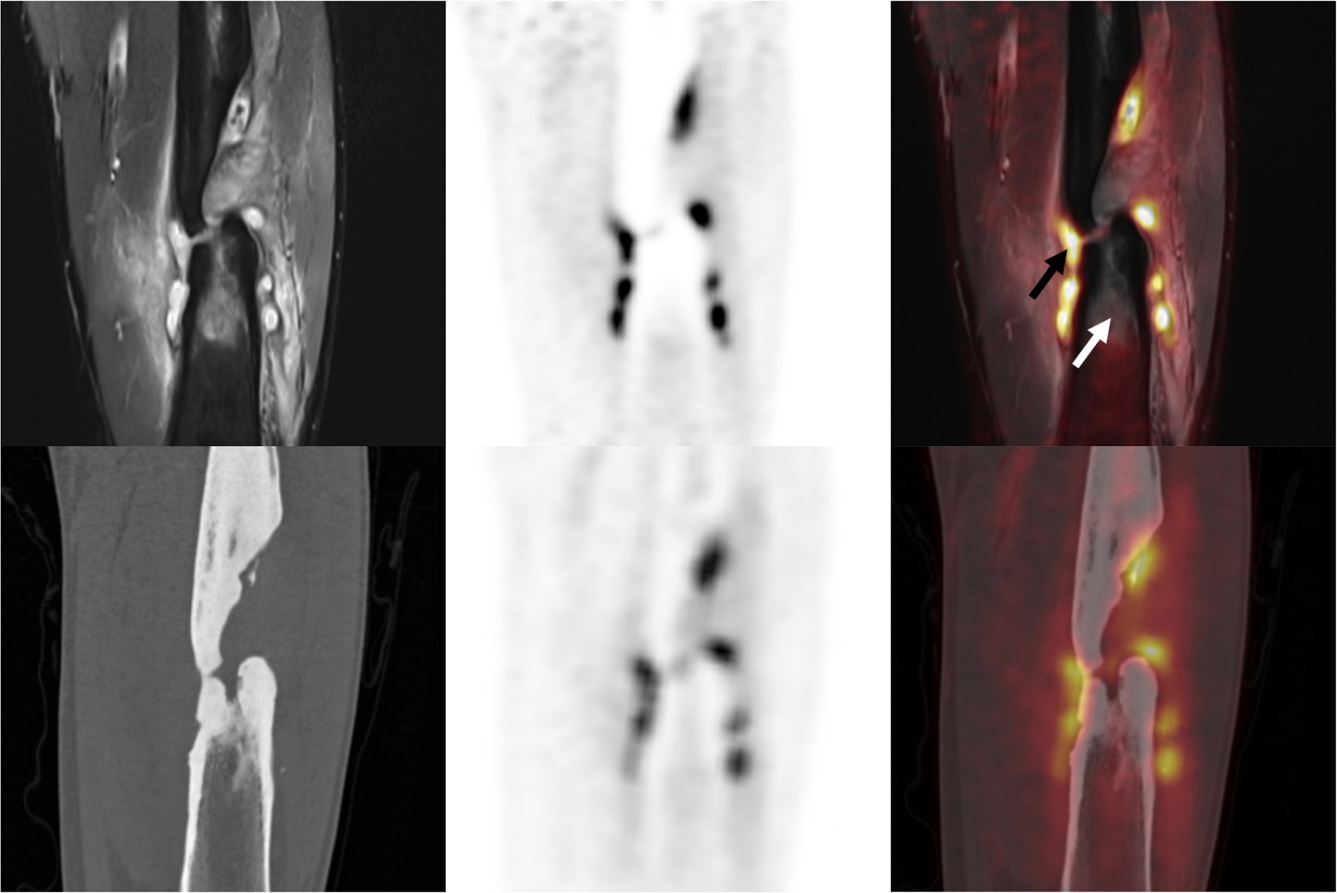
Reconstructed coronal images of the affected region (left femur) from patient 4. Top row, left to right: T2-weighted MRI, PET component of PET/MRI, and hybrid PET/MRI overlay. Bottom, from left to right: CT, PET component of PET/CT, and hybrid PET/CT overlay. Black arrow denotes PET/MRI is able to correlate the location of high FDG uptake with high intensity on T2-weighted MRI. White arrow denotes T2-weighted MRI shows intramedullary oedema that does not show increased FDG uptake. The features at both arrows are very influential information for a physician planning treatment
